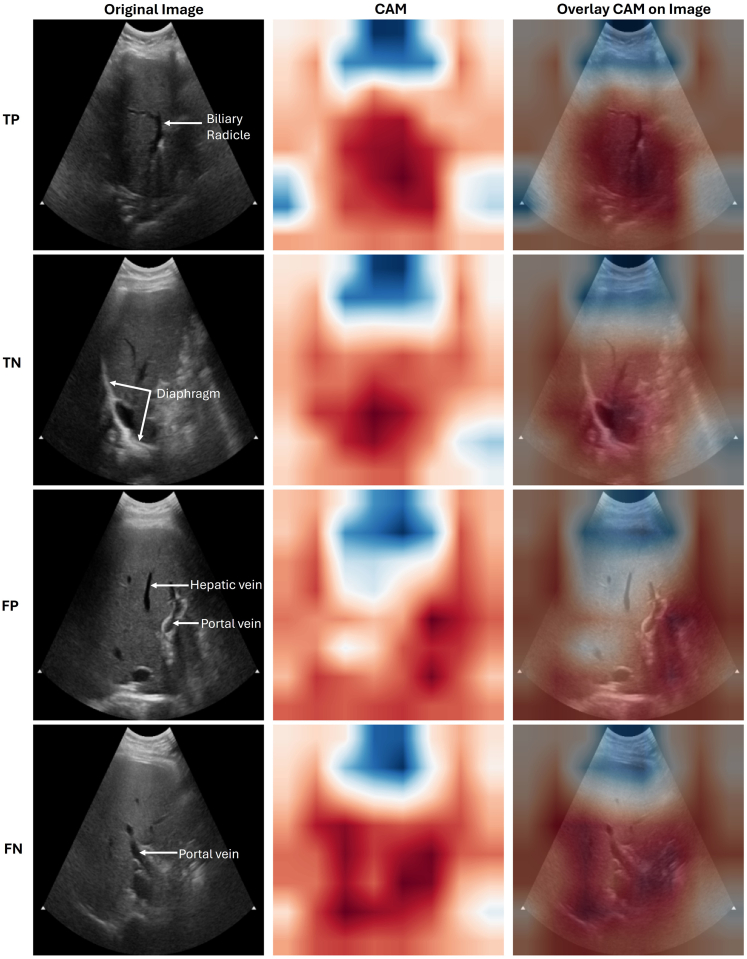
Class Activation Maps (CAM) for a randomly selected True Positive (TP), True Negative (TN), False Positive (FP), and False Negative (FN) prediction. The target class predicted is moderate-to-severe (S2/S3) HS. Red areas represent image regions that are most relevant for model prediction, with higher intensities (darker red) corresponding to higher importance. Similarly, blue areas represent regions that the model considers least relevant for prediction, with higher intensities corresponding to lower importance. Plots reveal that the model focuses on mid- and far-fields (the bottom 2/3) of the ultrasound image, primarily on the bulk of the hepatic parenchyma including the diaphragm and portal vasculature.
